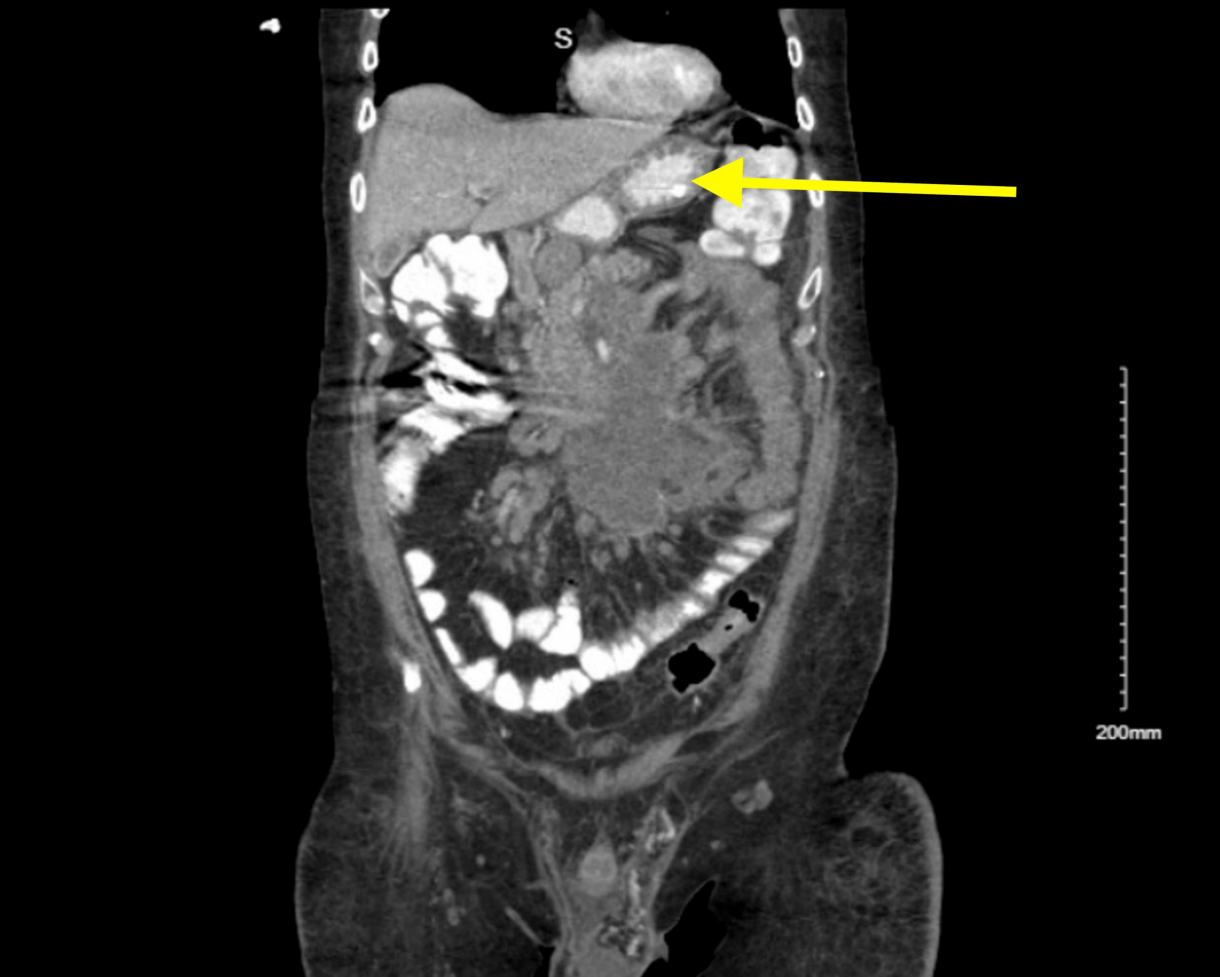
Coronal section of CT abdomen and pelvis The yellow arrow shows the normal-sized stomach with complete resolution of the gastric dilatation and gastric outlet obstruction after chemotherapy.
